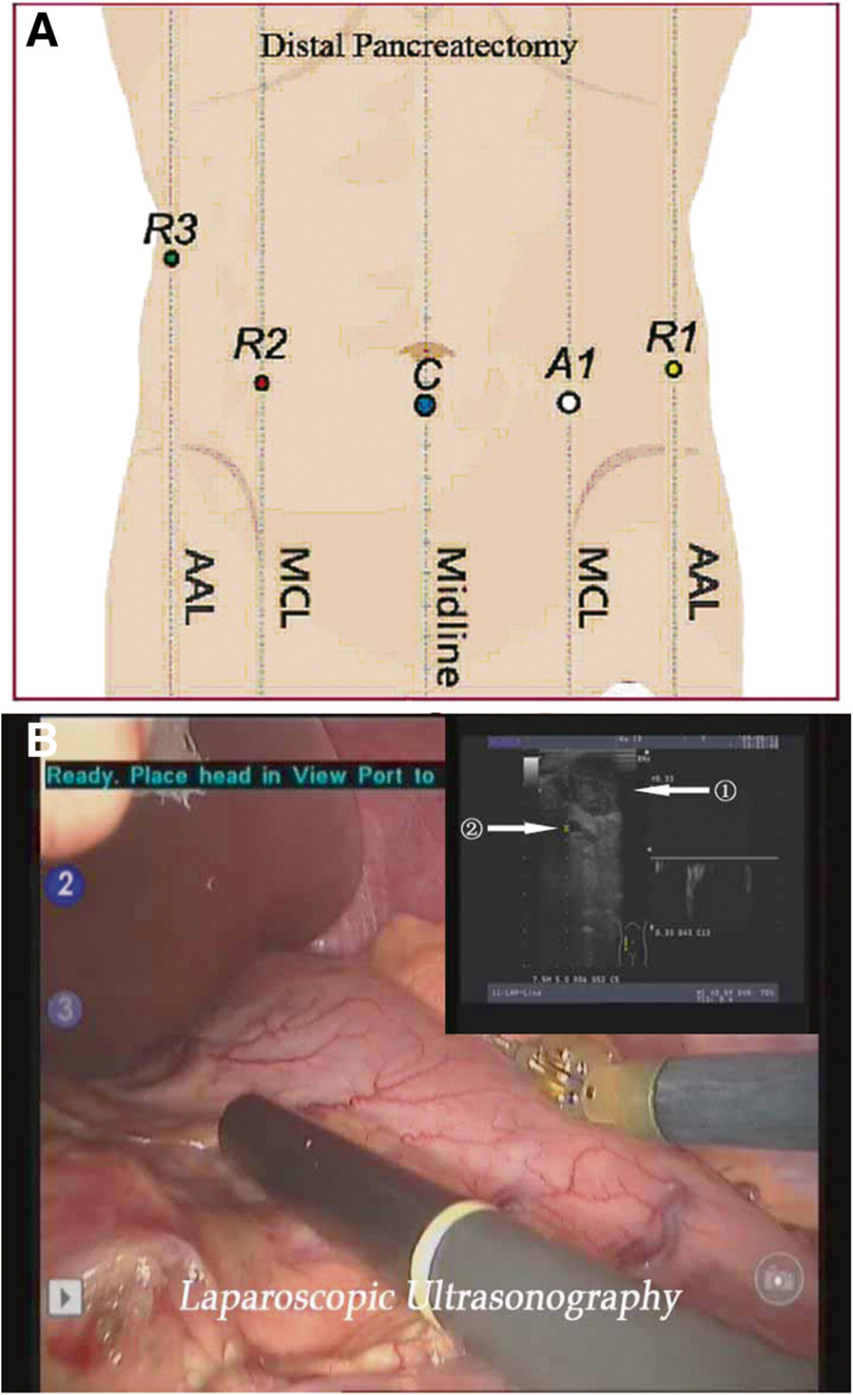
a Port placement for RDP. *C* camera port (12 mm), *R1* left robotic instrument port (8 mm), *R2* right robotic instrument port (8 mm), *R3* third robotic instrument port (8 mm), *A1* first assistant port (12 mm), *MCL* midclavicular line, *AAL* anterior axillary line. b Intraoperative laparoscopic ultrasound examination of the pancreas. *Arrow 1* shows the tumor. *Arrow 2* shows the splenic vessels
